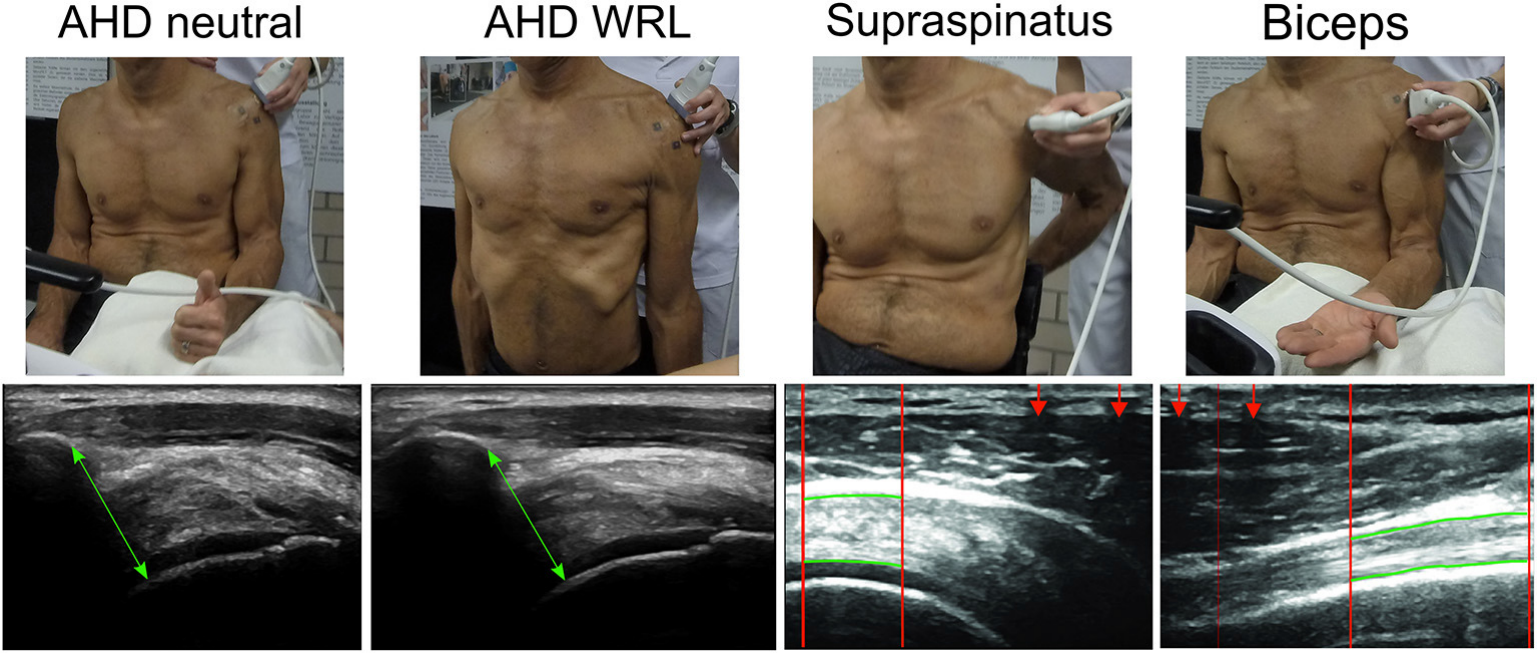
Ultrasound measurements: position and example of measurement. Each type of measurements represents a different example participant and does not relate to the person of the position images. AHD neutral: acromiohumeral distance images in the neutral position were taken in a seated position with 90° elbow flexion with the thumb facing upward. AHD WRL: acromiohumeral distance images during WRL. Supraspinatus: transverse ultrasound images of supraspinatus tendon were taken in a seated position with the palm placed on the lower back, shoulder extended, and the elbow flexed posteriorly. Biceps: longitudinal images of long head of biceps brachii tendon were taken in a seated position with 90° elbow flexion and the hand palm facing upward. The region of interest (ROI) is presented between the red vertical lines with the upper border and lower border of the tendons marked with the manually identified red horizontal lines. Lines are marked thicker as compared with the actual analysis for visualization reasons. ROI is selected based on the interference pattern that resulted from a metal marker taped to the skin (assigned with red arrows).
